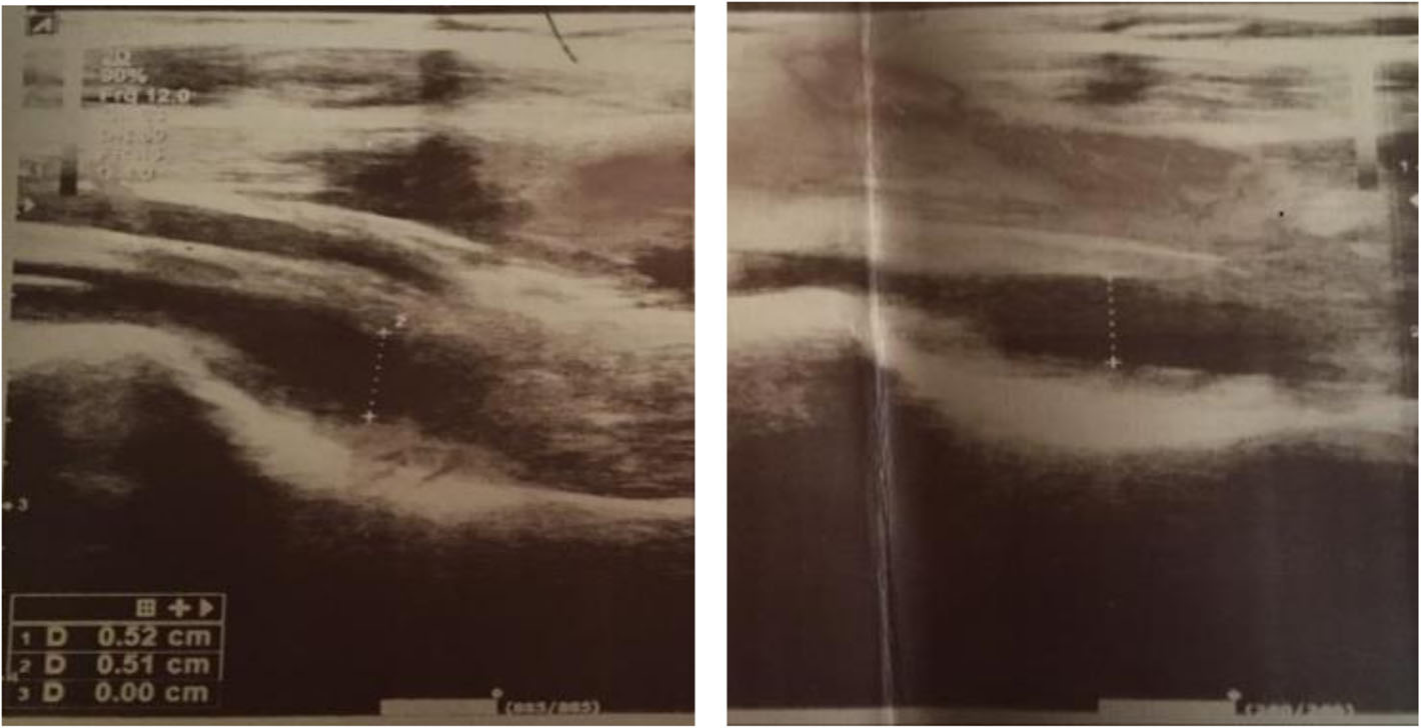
Ultrasound of both hip joints in the second patient. Bilateral mild hip joints effusion, especially in anterior synovial space of left side
